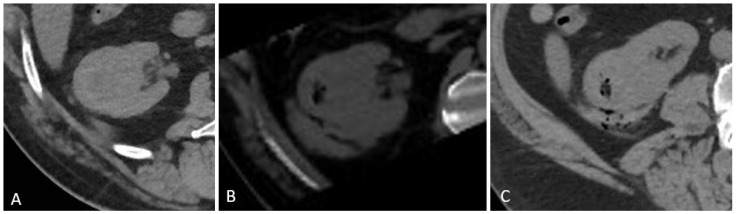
(A) Suspected renal mass—target to CT-guided percutaneous biopsy, (B) post-biopsy non-contrast CT scan, showing the dissemination of the gelatin foam paste as well as a small perirenal hematoma, which remained stable at the 4 h follow-up non-contrast CT (C).
